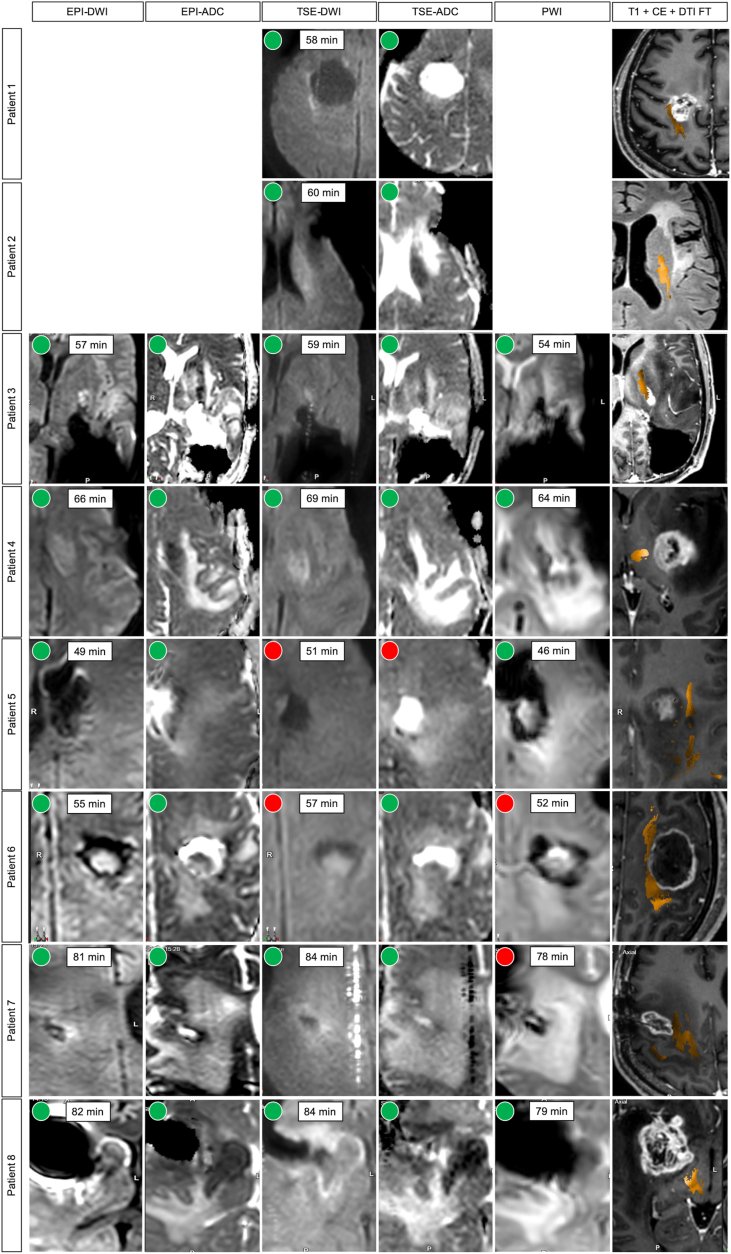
Detection of ischemia within the motor system in ioMRI. The figure shows the diffusion-weighted imaging (DWI) and perfusion-weighted imaging (PWI) sequences as well as the location of the corticospinal tract (orange) as visualized by diffusion tensor imaging fiber tracking (DTI FT) fused with preoperative T1-weighted images with contrast enhancement (T1 ​+ ​CE) of all patients who showed a loss or decline of motor evoked potentials (MEP) during tumor resection. Green circles show that ischemia could be detected by the according sequence. Red circles indicate that ischemia could not be detected. The intervals between the loss or decline of MEPs and the completion of the image is shown for each sequence in minutes (EPI ​= ​echo-planar-imaging, ADC ​= ​apparent diffusion coefficient, TSE ​= ​turbo-spin-echo, PWI ​= ​perfusion-weighted imaging). (For interpretation of the references to colour in this figure legend, the reader is referred to the Web version of this article.)
